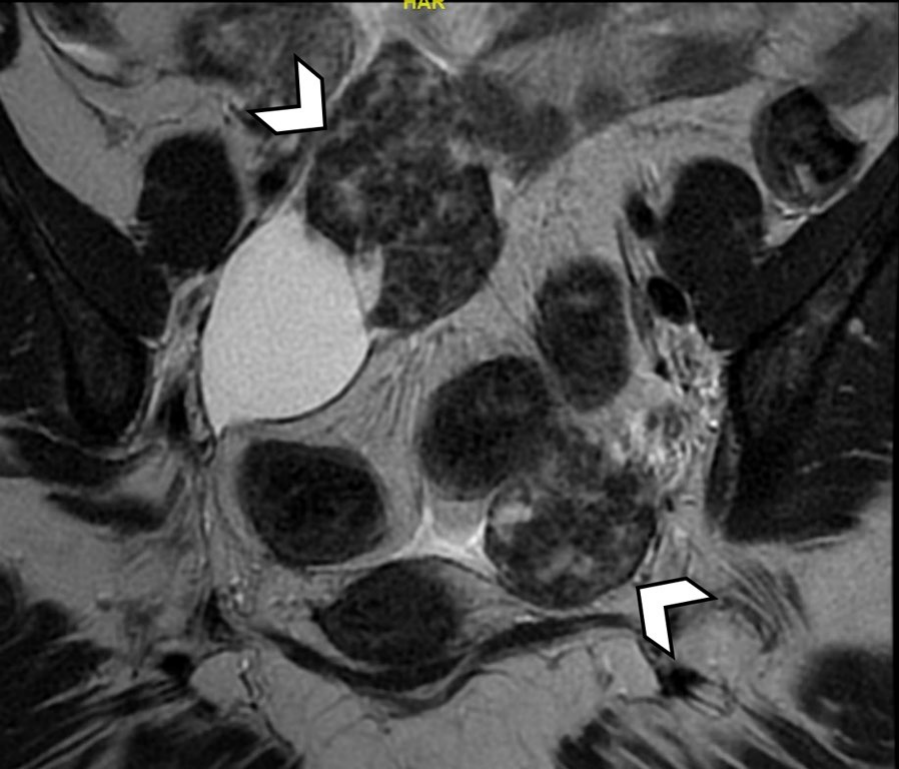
Bilateral solid ovarian masses first detected on staging CT in a 54-year-old female with newly diagnosed ILC. Coronal T2-weighted MR image of the pelvis shows bilateral, solid, ovarian masses with heterogeneous low T2-weighted signal (arrowheads), suggestive of fibrous, desmoplastic components. There is an ovarian cyst adjacent to the right ovarian mass. The solid appearance of the masses and low T2-weighted signal favor Krukenberg tumors over primary ovarian malignancy. Metastatic ILC involvement was confirmed following hysterectomy and bilateral salpingo-oophorectomy
